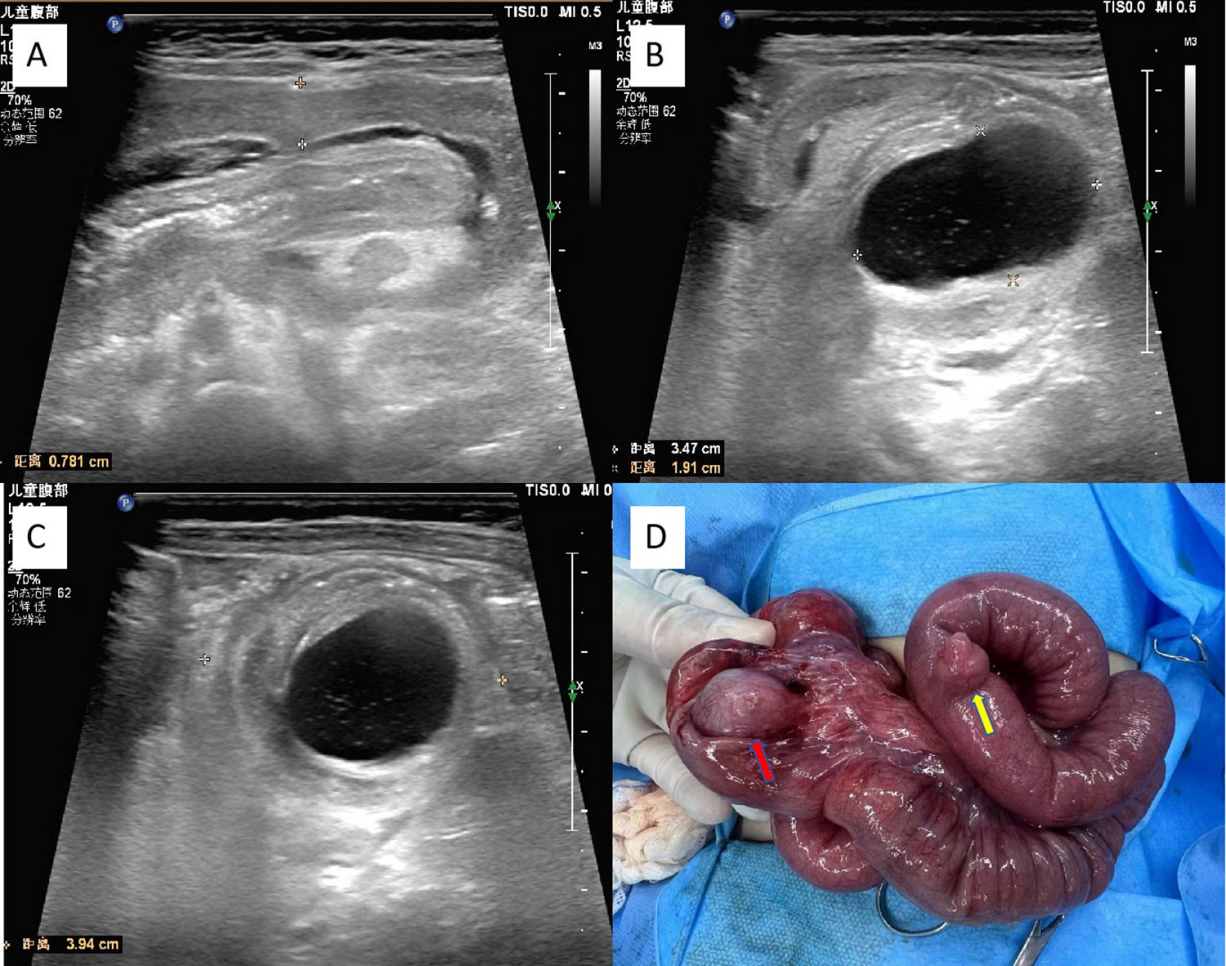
Preoperative color ultrasound and intraoperative images. (A) Abdominal ultrasound revealed intussusception, presenting a “sleeve sign” in the longitudinal section. (B) Abdominal ultrasound displays image of the cystic long axis of the intussusception head. (C) Abdominal ultrasound revealed the cystic long axis of the intussusception head. (D) Ileocecal intestinal duplication (Red arrow) and Meckel's diverticulum (Yellow arrow).
